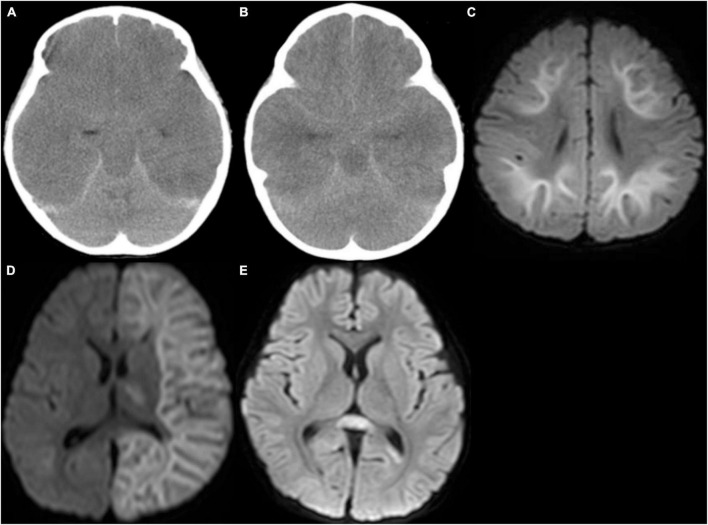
Computed tomography (CT) and magnetic resonance imaging. (A) CT in a patient with encephalopathy with fulminant acute cerebral edema revealed diffuse low density in the cerebrum with blurring of gray-white matter junction, and disappearance of ambient cistern, suggesting severe cerebral edema with probable downward herniation. (B) CT in a patient with hemorrhagic shock and encephalopathy syndrome revealed severe cerebral edema. (C) Diffusion-weighted image in a patient with acute encephalopathy with biphasic seizures and late reduced diffusion (AESD) revealed high signal lesion in the subcortical white matter with sparing peri-Rolandic regions, so-called bright tree appearance. (D) Diffusion-weighted image of a patient with unilateral AESD revealed left-sided bright tree appearance (BTA) with a small lesion in the left ventral thalamus. (E) Diffusion-weighted image of a patient with mild encephalitis/encephalopathy with reversible splenial lesion (MERS) revealed a high signal lesion in the splenium of the corpus callosum.
